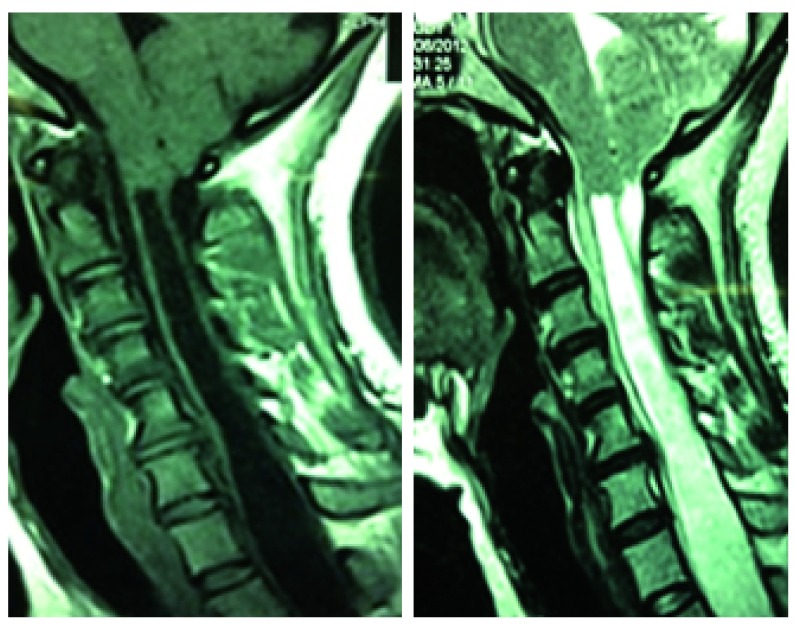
T1 and T2 MRI showing descended cerebellar tonsils and wide syrinx starting from C2.
